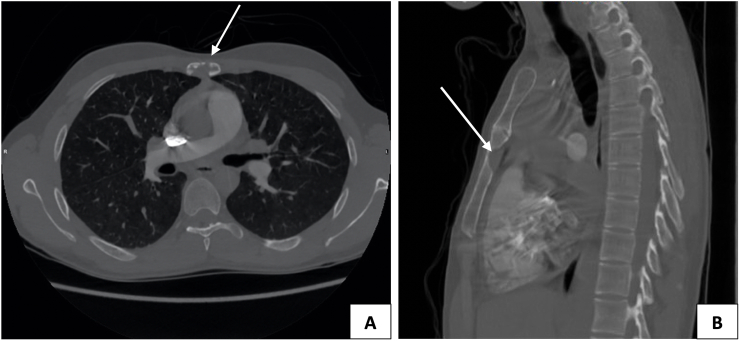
(A) Axial and (B) Saggital CT chest with a lytic lesion of the sternum in bone window.
